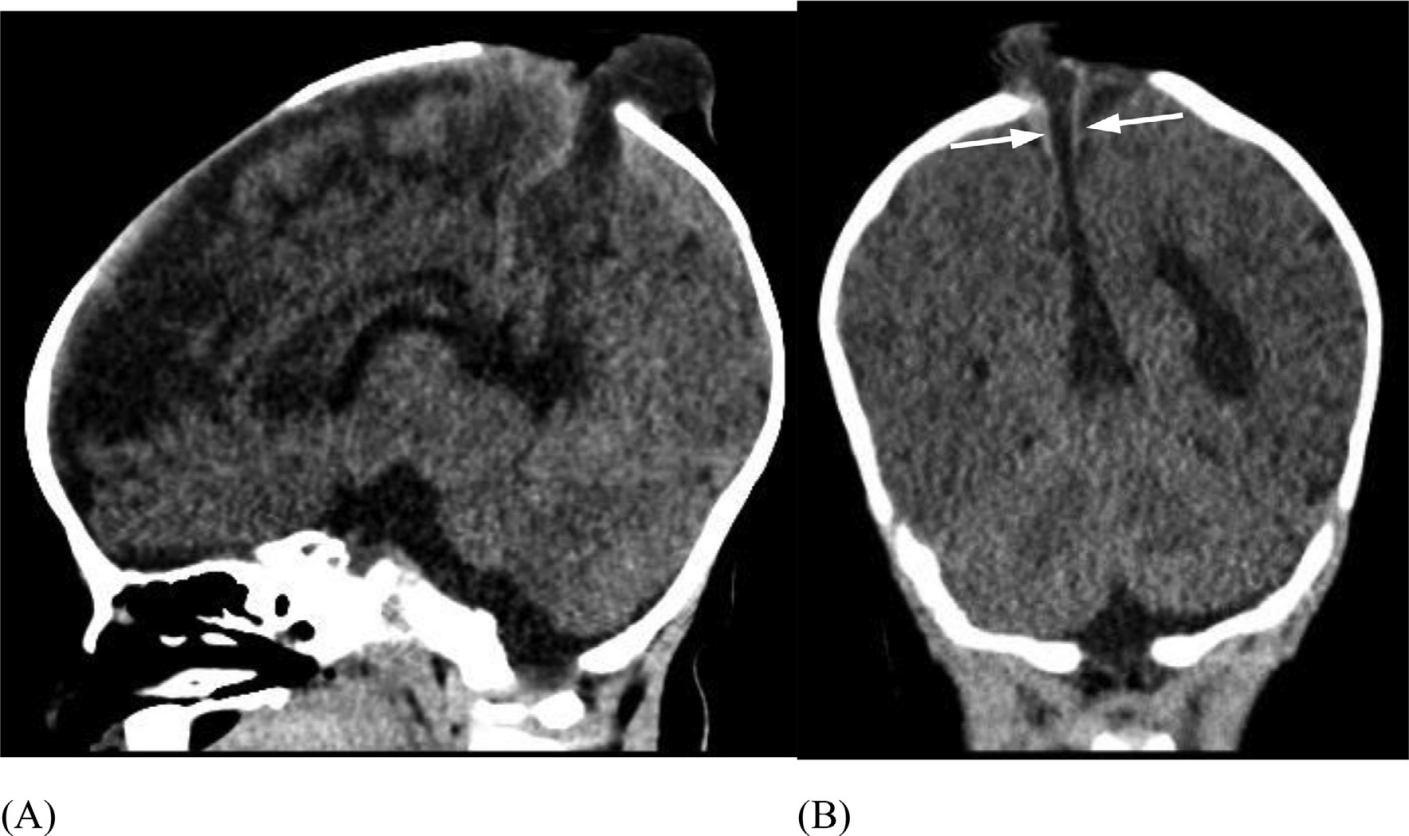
(Case 2) Sagittal midline CT (A) demonstrates the calvarial defect cranium bifidum with atretic parietal cephalocele. Coronal CT (B) demonstrates fenestration of the superior sagittal sinus at the atretic parietal cephalocele (arrows).
